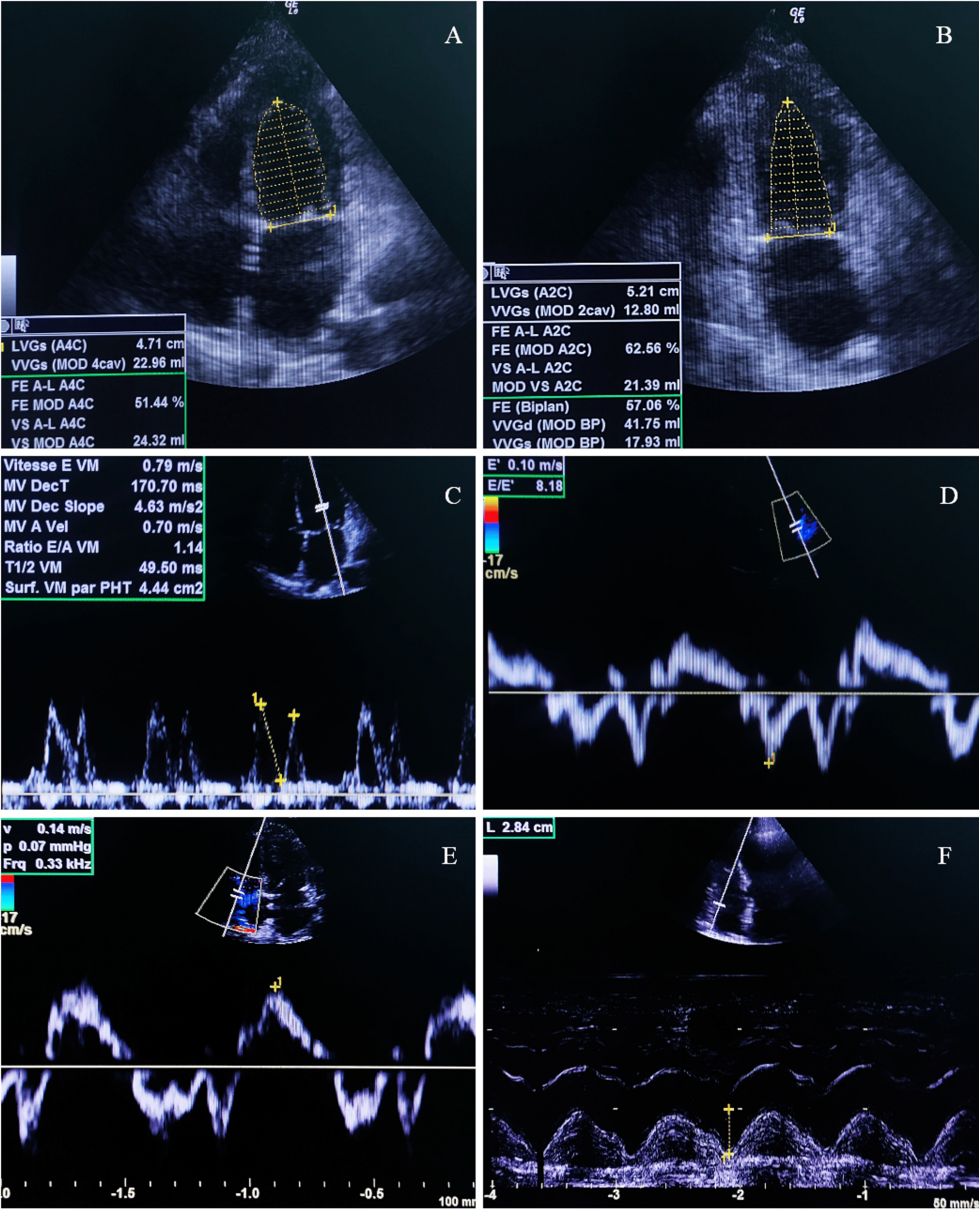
Transthoracic echocardiography performed on the seventh postoperative day (A) Four-chamber view in bidimensional mode and (B) two-chamber view mode both show complete normalization of left ventricular systolic function, LVEF: 57%. (C) Pulse-wave Doppler on the mitral funnel showing a normal transmitral filling pattern (E:79 cm/s, A:70cm/s E/A:1.14).
